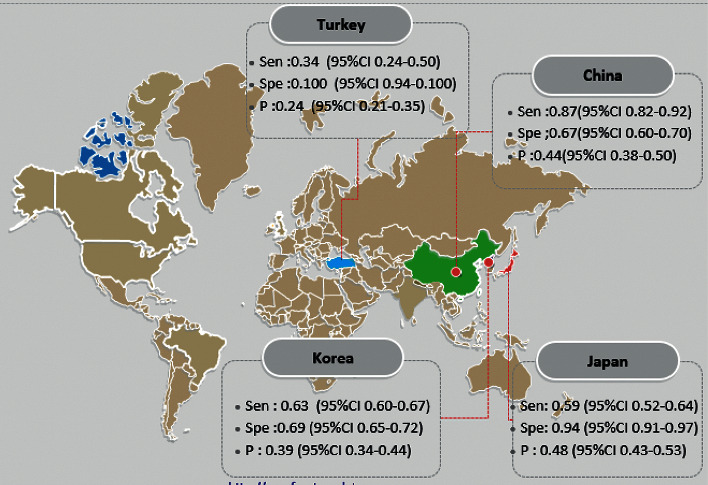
Meta-analysis of PET/CT in diagnosing lymph node metastasis in colorectal cancer based on country.
